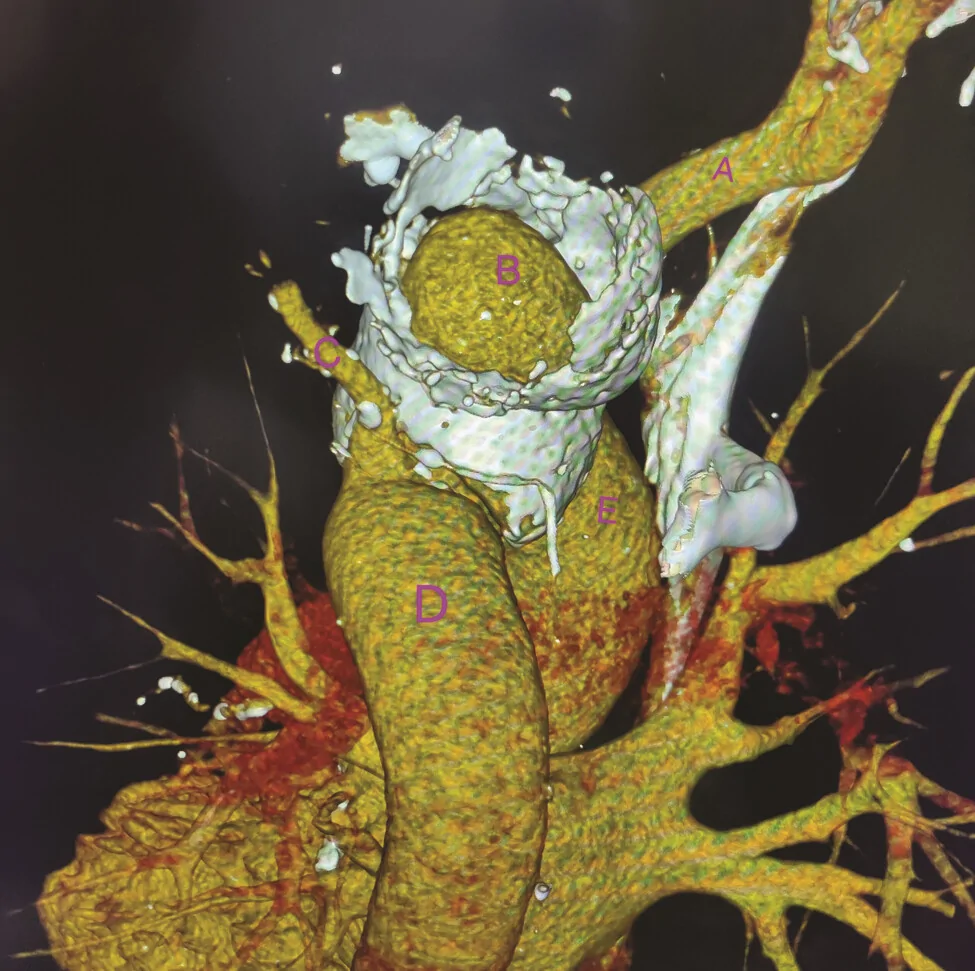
Volume-rendered three-dimensional CT reconstruction—the calcified aortic arch pseudoaneurysm and its relation to the arch vessels. Labels: A, innominate artery; B, pseudoaneurysm; C, left subclavian artery; D, distal arch; E, ascending aorta. CT, computed tomography.
